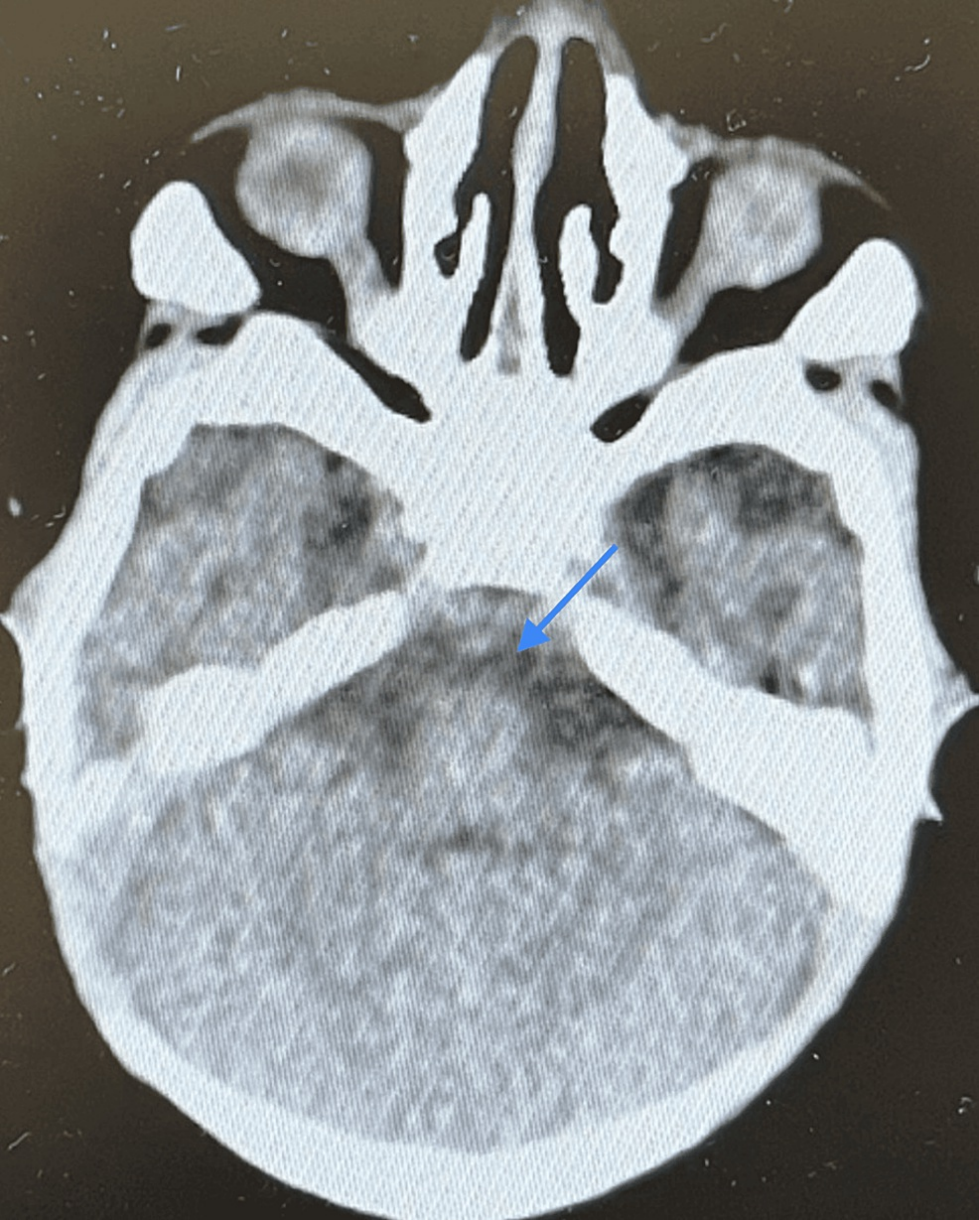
Non-contrast CT brain: posterior fossa structures degraded.
